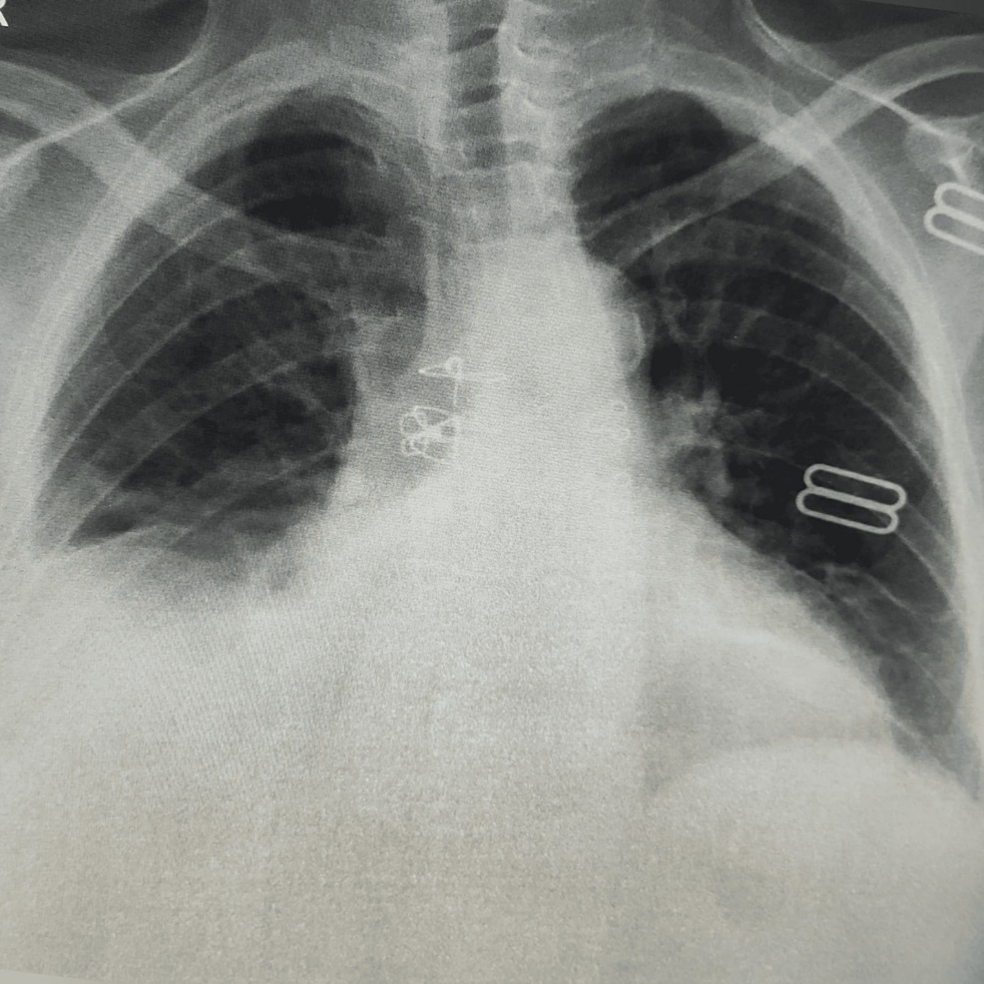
Chest X-ray on admission showing right pleural effusion
